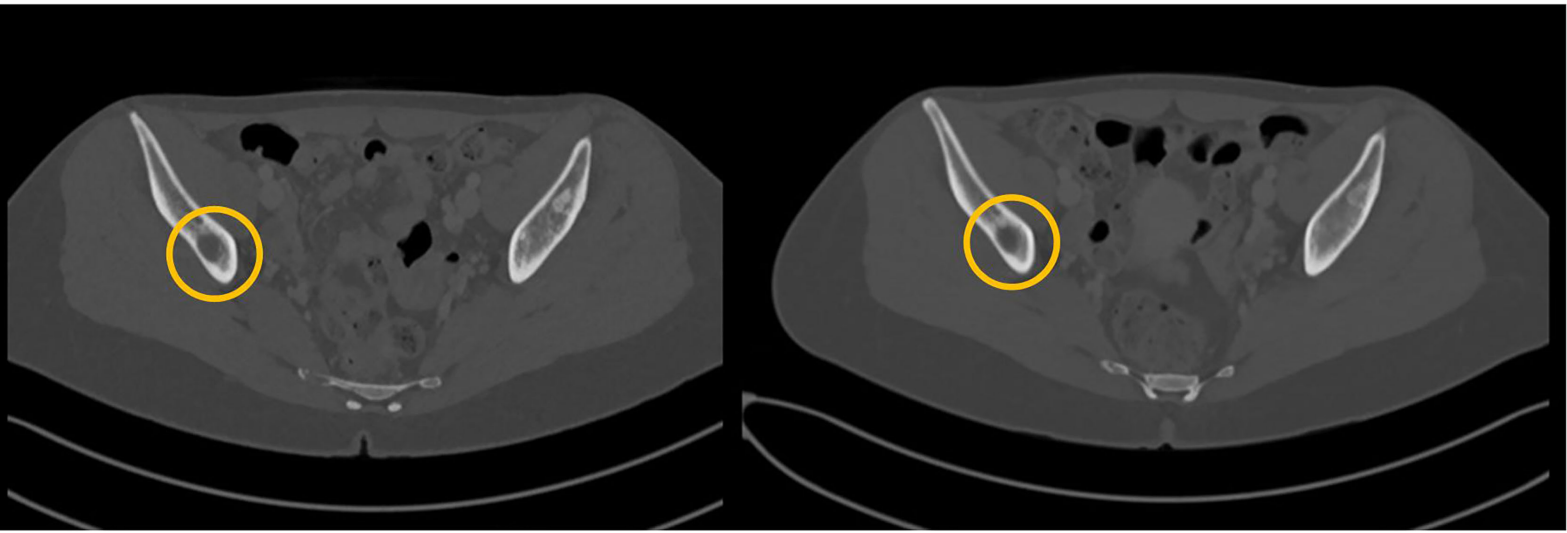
After 14 months from denosumab discontinuation due to AFF, progressive disease was documented (new lesions in right iliac wing and L5 soma, left panel) and denosumab restarted. On the right panel, a CT scan obtained 3 months later shows stabilization of disease (stable disease according to RECIST 1.1).
